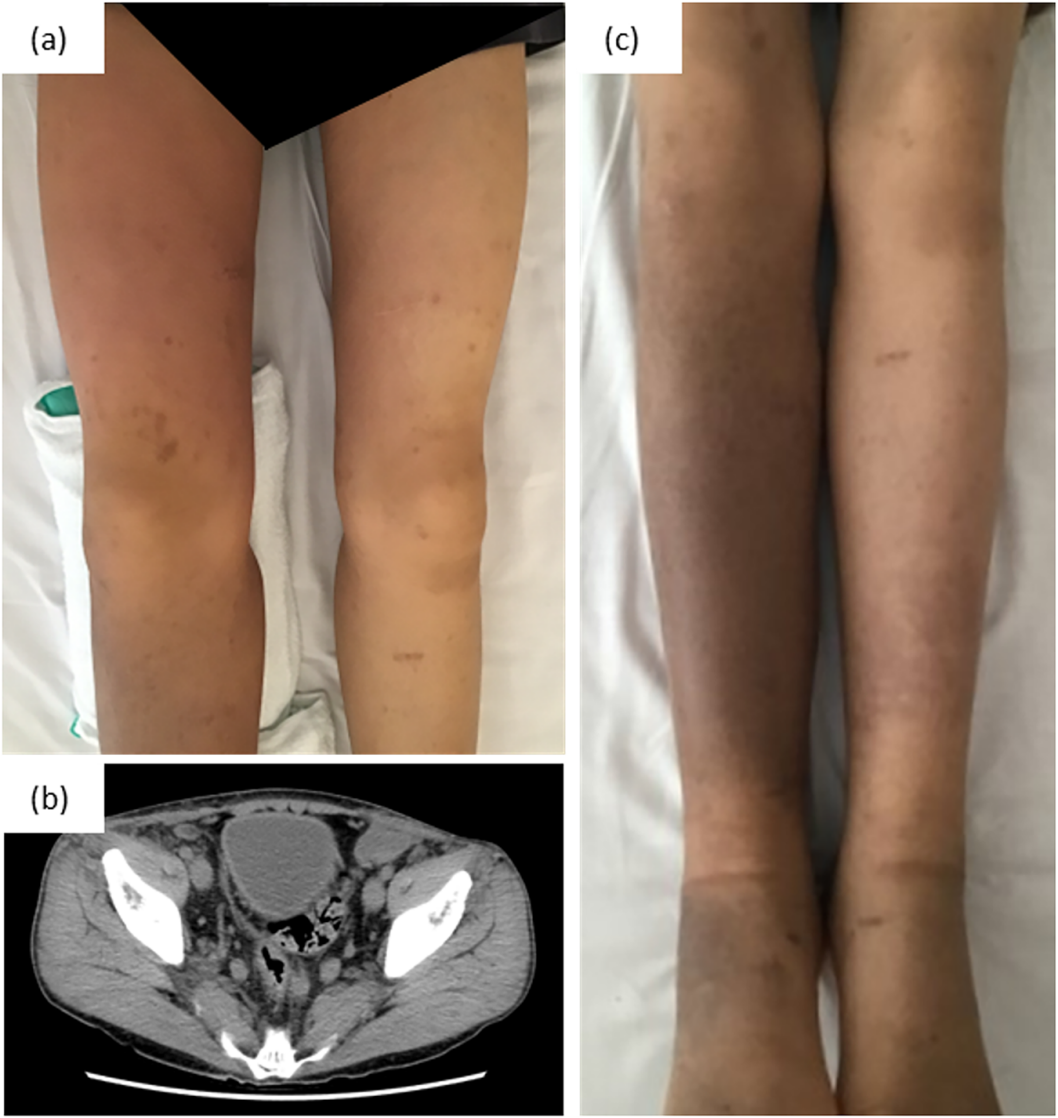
Clinical features of patient 1. a Physical examination reveals bilateral lower limb swelling, more severe in the right leg, with accompanying redness extending to the right inguinal region. b Computed tomography showing multiple enlarged pelvic lymph nodes. c Swelling and redness improved after antibacterial treatment.
